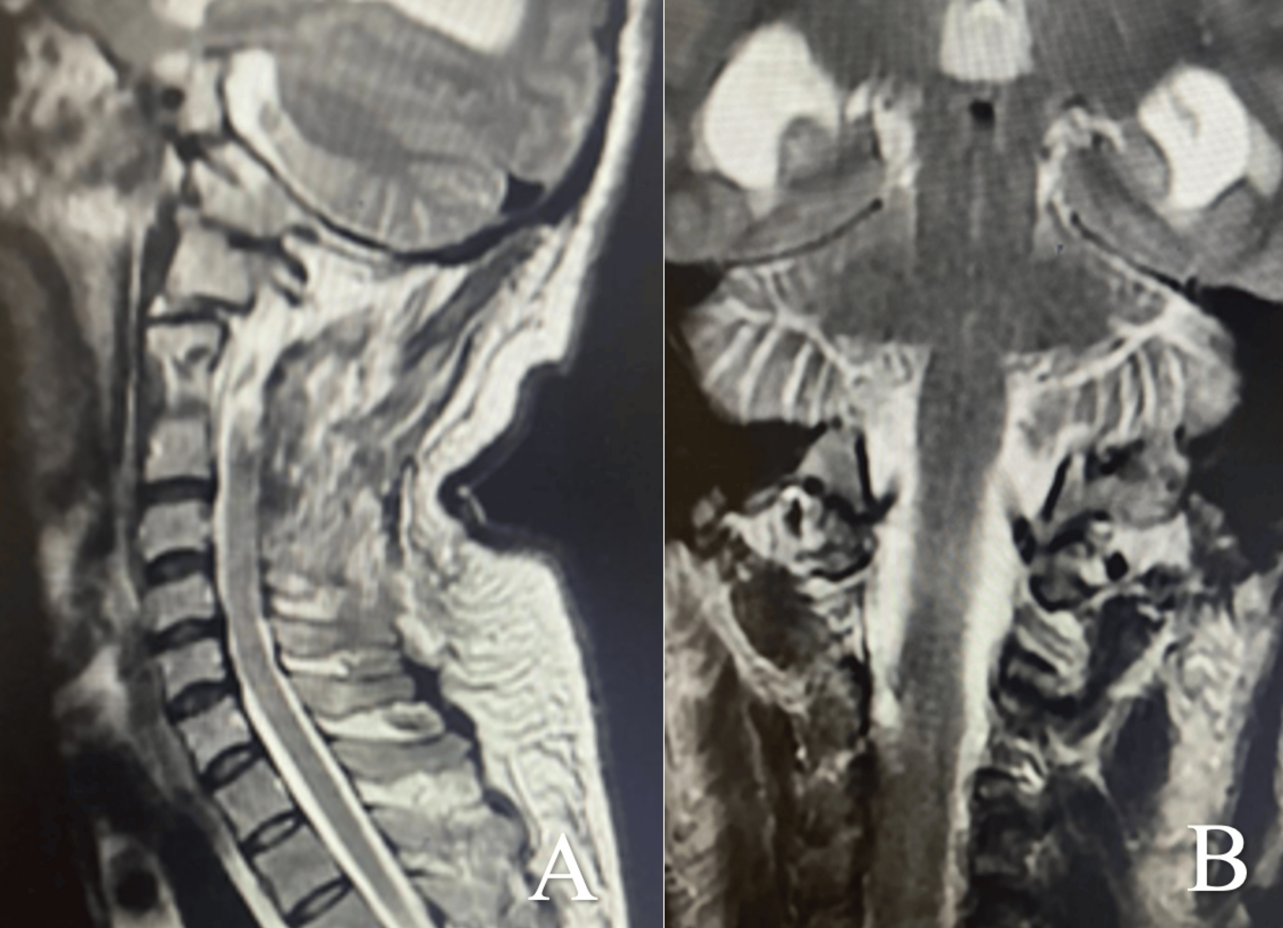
Cervical MRI: postoperative T2-weighted images, sagittal (A) and coronal (B) planes. Postoperative imaging confirmed the total resection of the intradural extramedullary cavernous malformation cavernoma.
